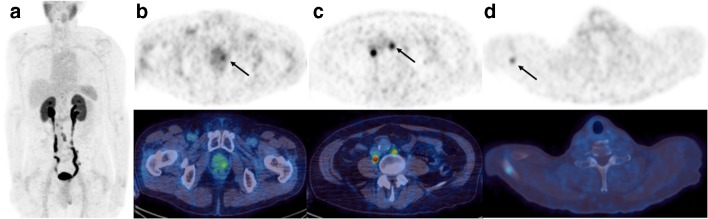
^68^Ga-THP-PSMA PET-CT scan of a man diagnosed with high-risk prostate cancer. a Maximum intensity projection image. b The primary tumour shows focal activity in the left peripheral zone (arrow). c There is evidence of retroperitoneal nodal disease (arrow) and d bone metastases (arrow). The detection of metastatic disease changed management from a surgical to systemic therapy approach with hormones and chemotherapy
